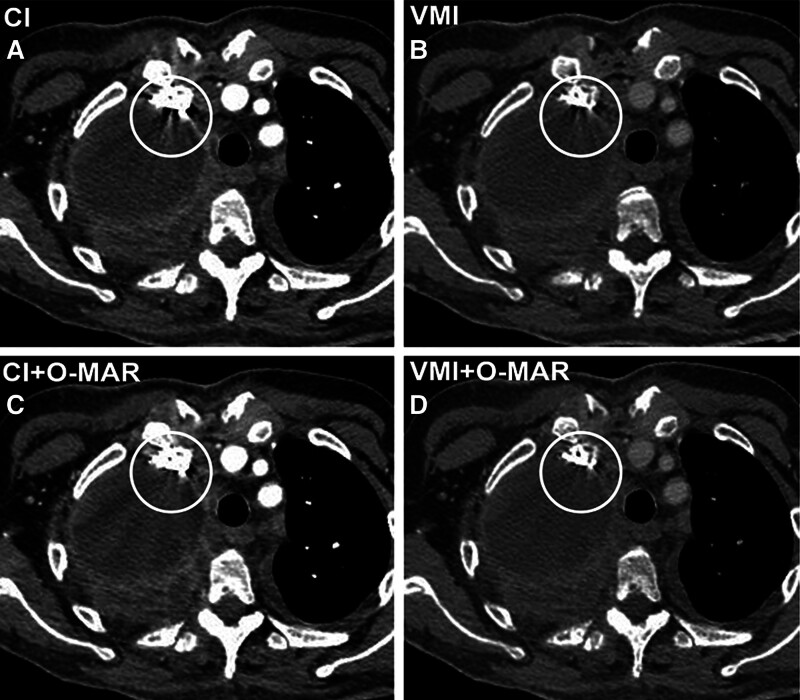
A 65-year-old woman with lung cancer. Enhanced chest CT images show pleural effusion and pleural thickening (circles). Artifacts caused by CM in the superior vena cava are the most severe on CI (A). VMI (B), and CI + O-MAR image (C) reduced some artifacts. VMI + O-MAR image (D) obtains the best subjective assessment scores from both reviewers. CI = conventional images, O-MAR = orthopedic metal-artifact-reduction, VMI = virtual monoenergetic images.
